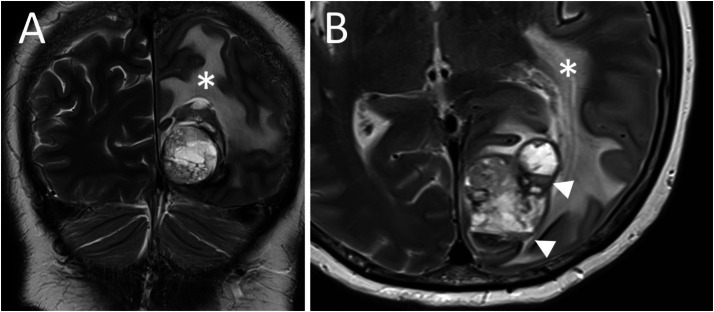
T2-weighted coronal (A) and axial (B) MRI images showing extensive perilesional oedema (star) and a multiloculated cystic occipital mass with fluid-fluid levels (arrowheads).
